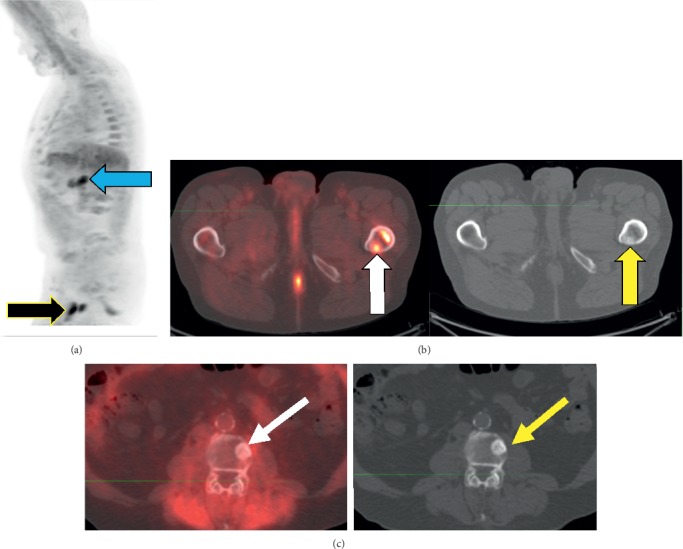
Images of a 79-year-old male who had undergone radical prostatectomy, salvage radiation, and proton beam therapy for oligometastases to L3 and multiple lymph nodes. He was referred for ^18^F-fluciclovine imaging with PSA rising to 2.03 ng/mL. (a) MIP view, sagittal plane. The black arrow points to enhanced uptake, consistent with metastasis in the proximal left femur. The blue arrow points to the pancreas (normal physiologic uptake). (b) Axial, fused PET/CT image (white arrow) and corresponding CT view (yellow arrow) show a metastasis in the proximal left femur. (c) Of interest, this PET/CT view and the corresponding CT view show a previously-irradiated metastasis in L3; note the absence of ^18^F-fluciclovine uptake (white arrow) on PET and presence of sclerosis on CT (yellow arrow).
